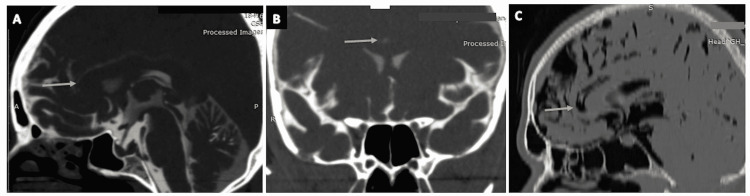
The cisterna pericallosa (pericallosal cistern). (A) and (B) show the pericallosal cistern (arrows) as seen on the sagittal and coronal sections of CT cisternography, respectively in a case of suspected CSF rhinorrhoea. (C) The pericallosal cistern (arrow) as seen on the sagittal section of CT brain (negative contrast) in a case of pneumocephalus.
